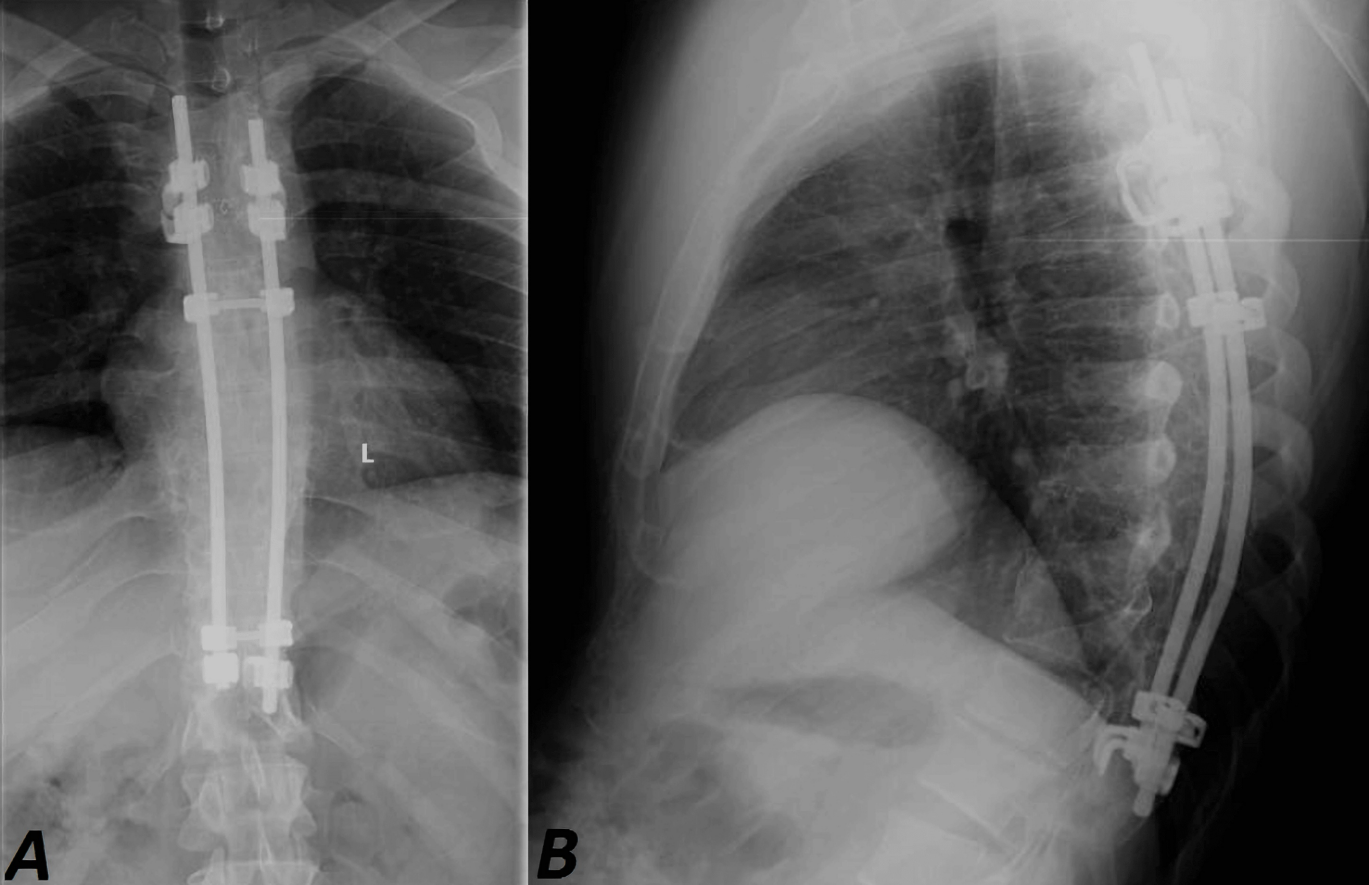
(A) Anteroposterior and (B) lateral radiographs show bilateral sublaminar wiring with proximal and distal claw hook stabilization from T4 to T10.
